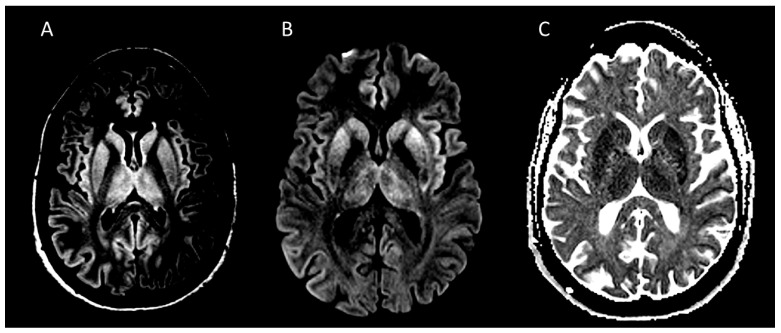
MRI findings in a patient with iatrogenic Creutzfeld-Jacobs Disease. (A): Axial FLAIR MRI image showing bilateral hyperintense signals in the basal ganglia, thalami, and cortical insular and cingulate ribbons, with asymmetric involvement of the right temporo-occipital cortex; (B): Diffusion-weighted imaging (DWI) demonstrates marked hyperintensity along the cortical ribbon, bilateral putamina, caudate nuclei, and thalami, consistent with restricted diffusion due to prion-induced spongiform change; (C): apparent diffusion coefficient (ADC) map reveals signal hypointensity in the same areas, confirming true restricted diffusion and excluding the T2 shine-through effect. The original images are stored at the Institute of Neurology of Southern Switzerland (INSI, Ospedale Civico di Lugano).
